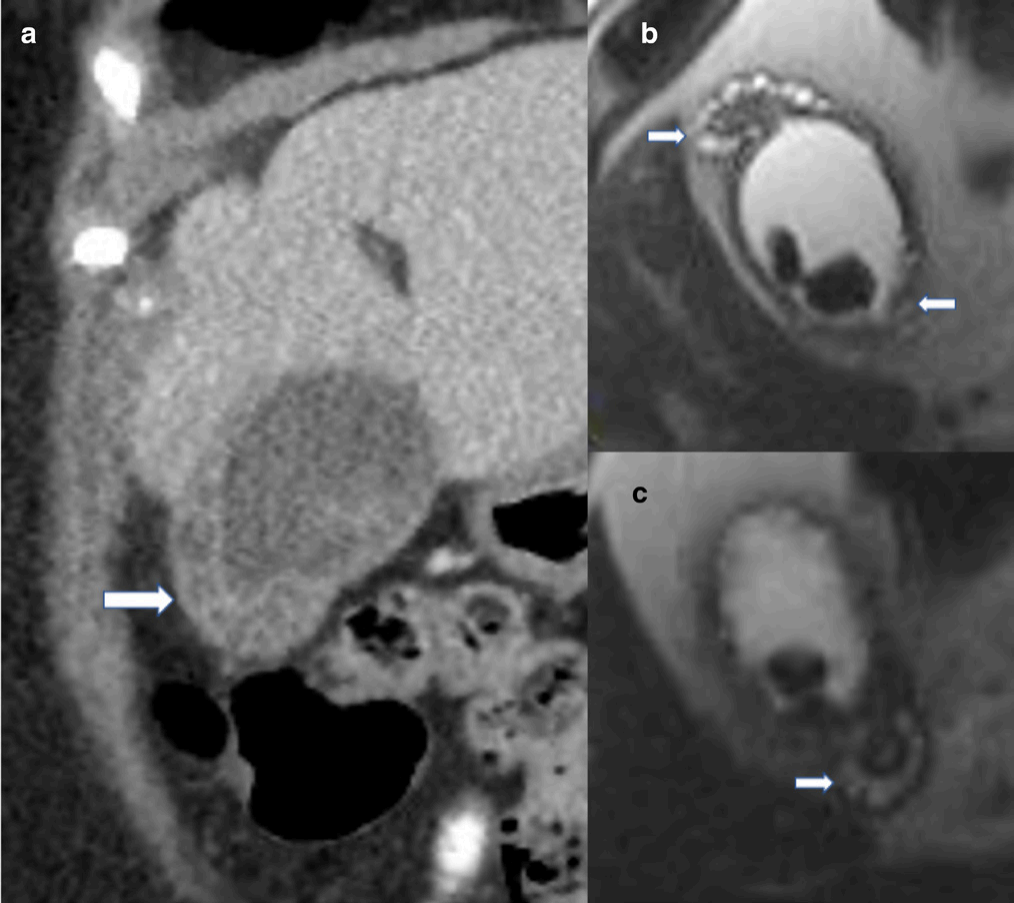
(a) Coronal contrast-enhanced CT, and (b, c) axial T2-HASTE MRI demonstrating classic “rosary bead” or “string of pearls” sign indicating the presence of Rokitansky-Aschoff sinuses within the thickened gallbladder wall seen in adenomyomatosis. Note the string of pearls appearance extends to involve the cystic duct in (c).
